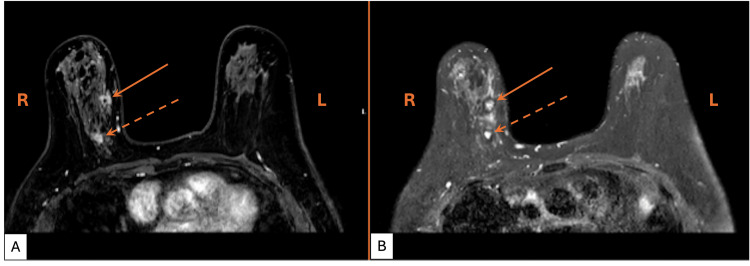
Diagnostic breast MRI showing two suspicious masses A) T1-weighted and B) T2-weighted axial breast magnetic resonance images. R, right side of patient; L, left side of patient. Solid arrow: there is an irregular, heterogeneously enhancing mass with spiculated margins measuring 28 x 19 x 11 mm at the 1 o'clock position in the upper inner quadrant of the right breast, 58 mm from the nipple-areolar complex. Dashed arrow: there is a second mass measuring 11 mm in the longest dimension at the 2 o'clock position, approximately 21 mm posterior and inferior to the index lesion. There is a non-mass enhancement extending between these two lesions spanning a total anterior-posterior extent of 50 mm.
